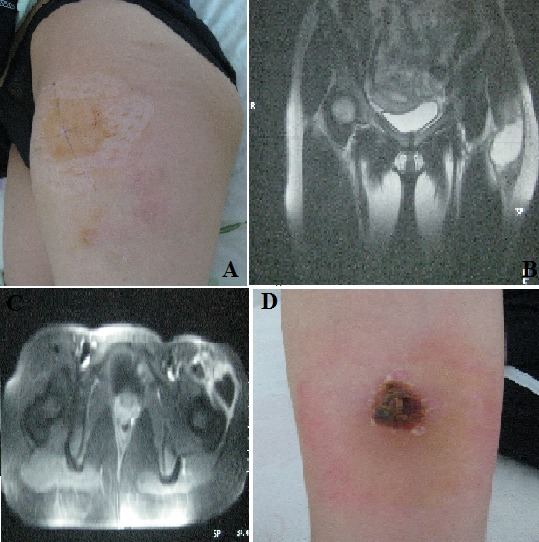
(A) swelling of the left anterior-external surface of the left thigh; (B, C) MRI of the hips: intra-muscular collection of the antero-lateral side of the thigh root, taking contrast in the periphery, involving the quadriceps muscle and tensor of facia lata; (D) tuberculin skin test positive with a phlyctenular appearance
